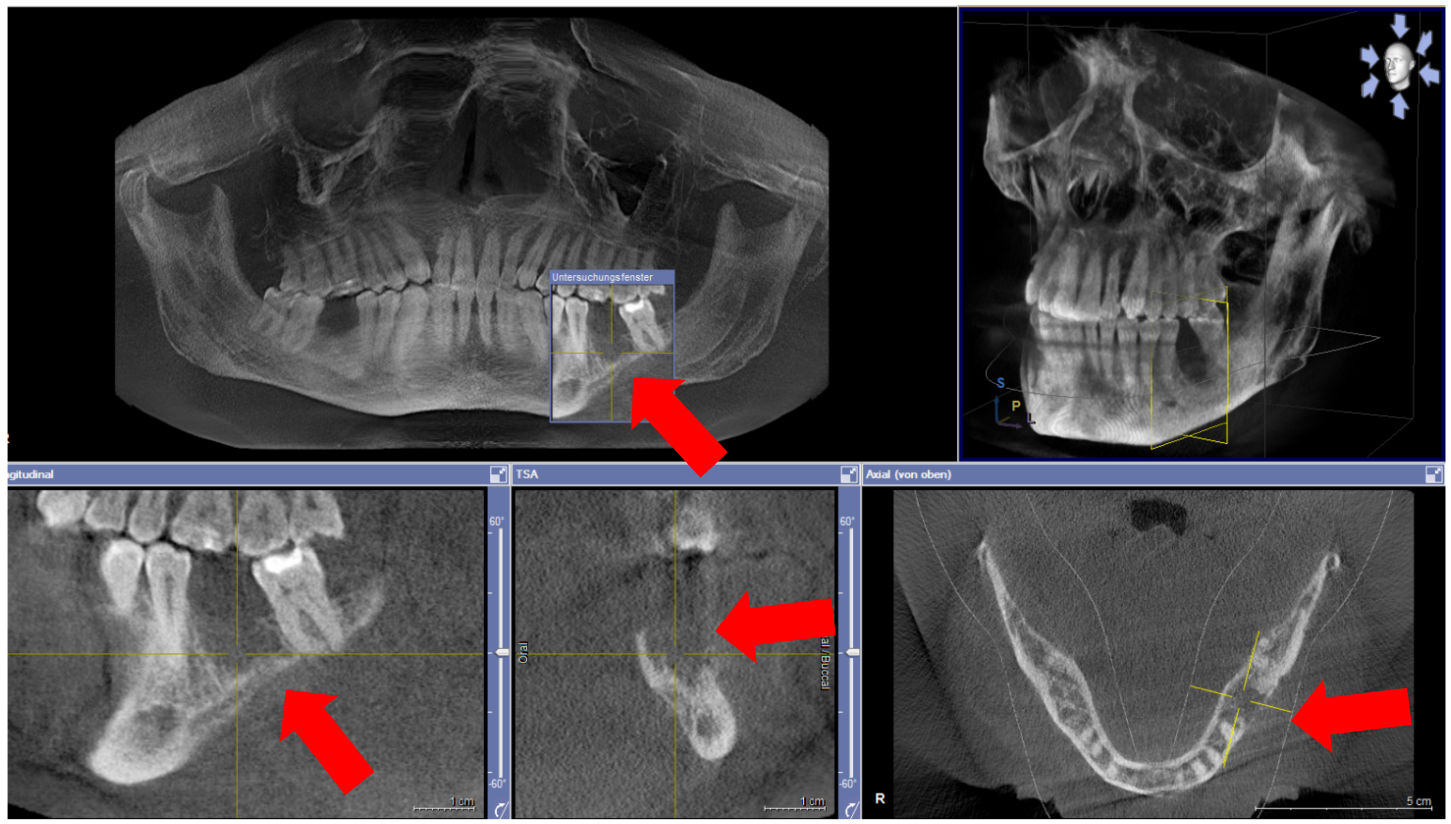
Cone Beam Computed Tomography (CBCT) with display of subsequent osteonecrosis of the lower left mandible in region 036 (red arrows)
